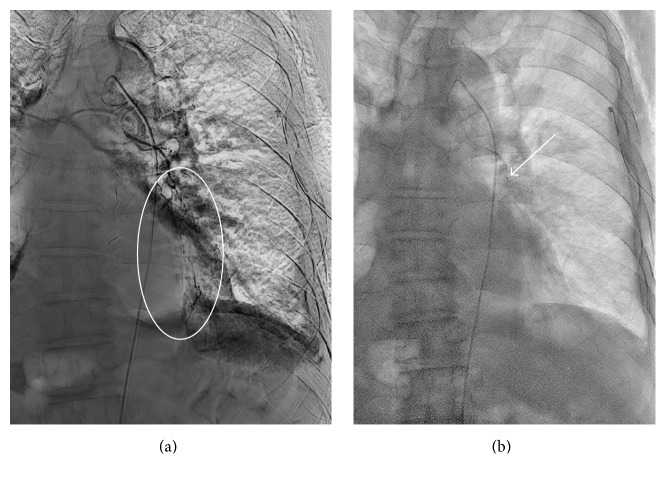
(a) A chest X-ray showed bleeding from the left bronchial artery in the left upper lobe (white circle). (b) A chest X-ray indicated the arrest of bleeding after bronchial artery embolization (white arrow).
